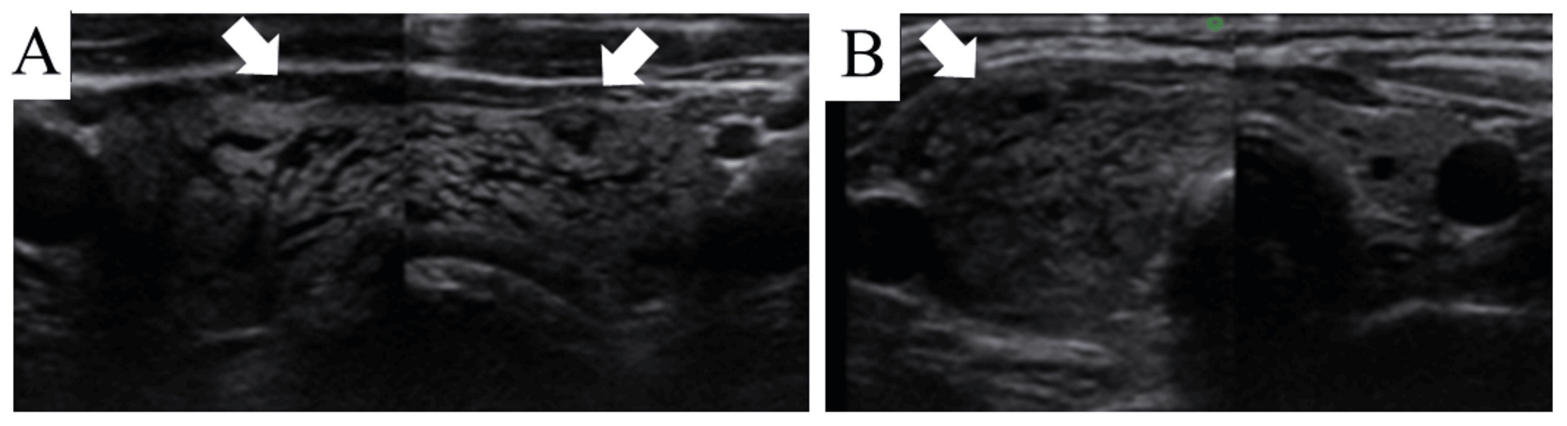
Ultrasound images of the thyroid glands. Bilateral (A) and unilateral (B) thyroid swelling after FNAB with typical ATTS features of hypoechoic cracked lesions. The white arrows depict the swollen thyroid glands. ATTS: acute transient thyroid swelling; FNAB: fine-needle aspiration biopsy
